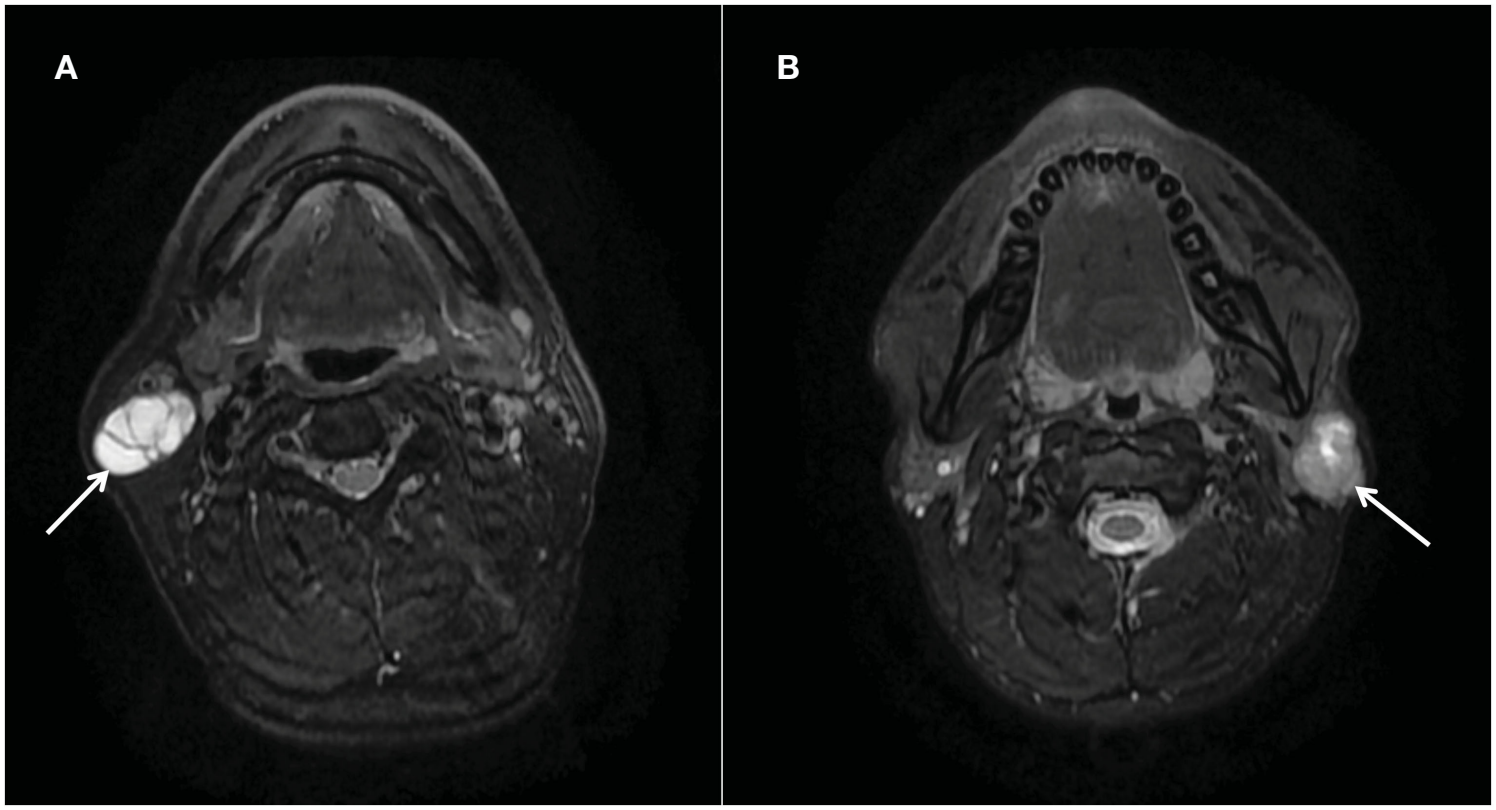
(A) Warthin tumors (arrows) in the right parotid gland of a 52-year-old man. T2-weighted image (axial plane) shows a markedly high-intensity tumor; a partition is visible within it. (B) Pleomorphic adenoma (arrows) in the left parotid gland of a 26-year-old man. T2-weighted image (axial plane) shows a slightly hypointense tumor. There are irregular areas of high intensity in the upper part of the tumor.
